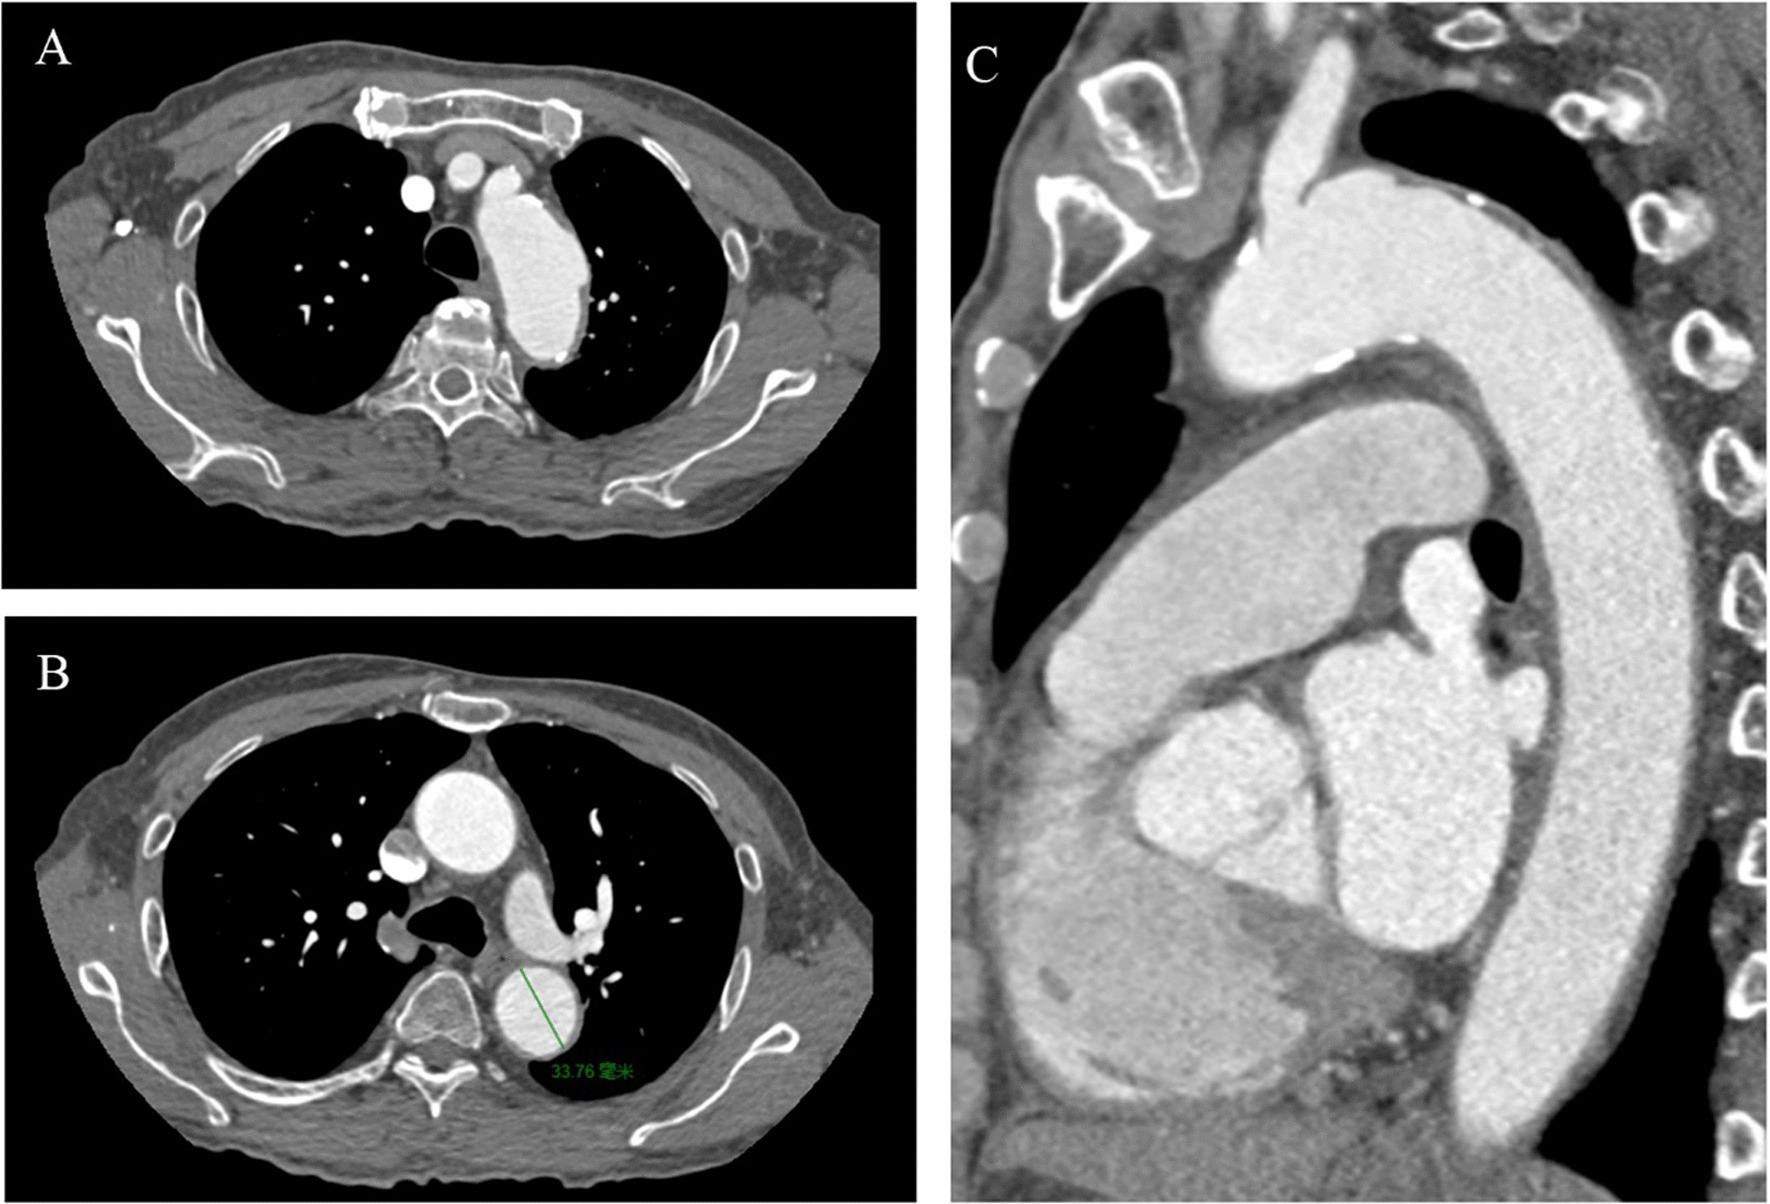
The contrast-enhanced computed tomography image shows the hematoma was significantly resolved and the ULP on the aortic arch disappeared after three months. A The transverse section of the aortic arch. B The section of the maximal aortic diameter (line). C Sagittal contrast-enhanced computed tomography shows resolved intramural hematoma of the descending aorta
